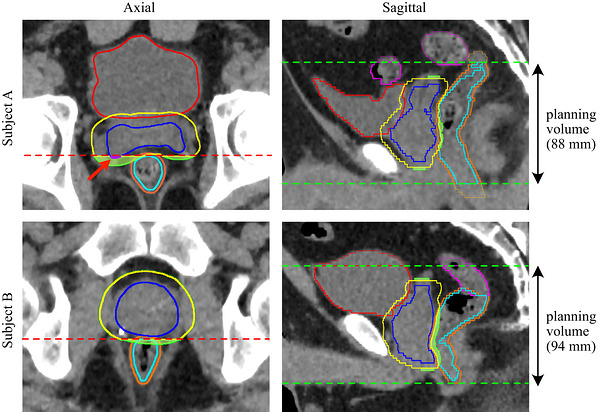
Axial and sagittal CT images of two representative subjects with delineated contours. Red and green dotted lines represent a lateral cut line passing through the anterior edge of the rectum‐2mm contour and planning volume boundary, respectively. The OAR contours outside the planning volume were also delineated with thin solid lines for visualization purposes. The purple area pointed out by a red arrow indicates the trimmed CTV volume with the lateral cut line. The green area is the subtracted volume of the PTV2 from the PTV1. Blue = CTV, green = PTV1, yellow = PTV2, orange = rectum, cyan = rectum‐2mm, red = bladder, magenta = sigmoid colon.
